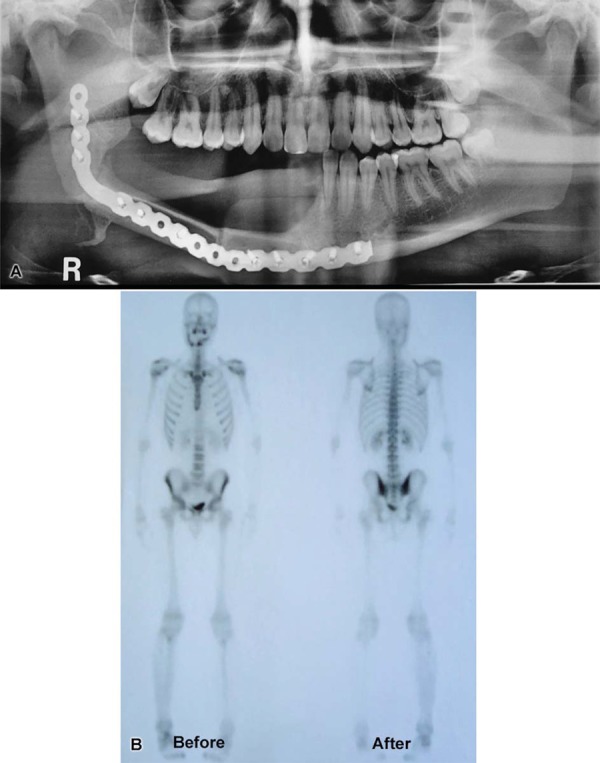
Panoramic radiograph (A) showing the reconstruction of the right mandible after surgical treatment. At the follow-up one year later bone scintigraphy (B) showed no signs of bone metastases
